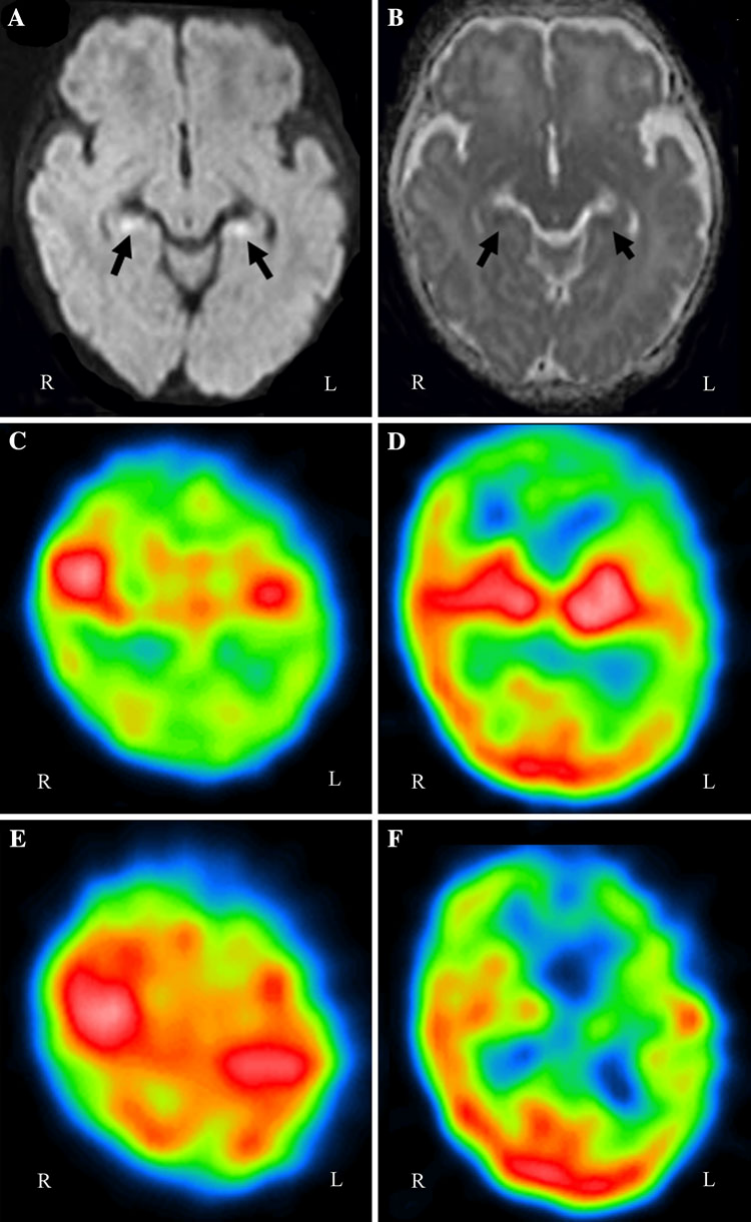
Magnetic resonance imaging (MRI) on day 6. Diffusion-weighted imaging (DWI) (a) revealed bilateral high-intensity regions in the hippocampus, and the apparent diffusion coefficient (ADC) (b) was low in the same regions. c–f
